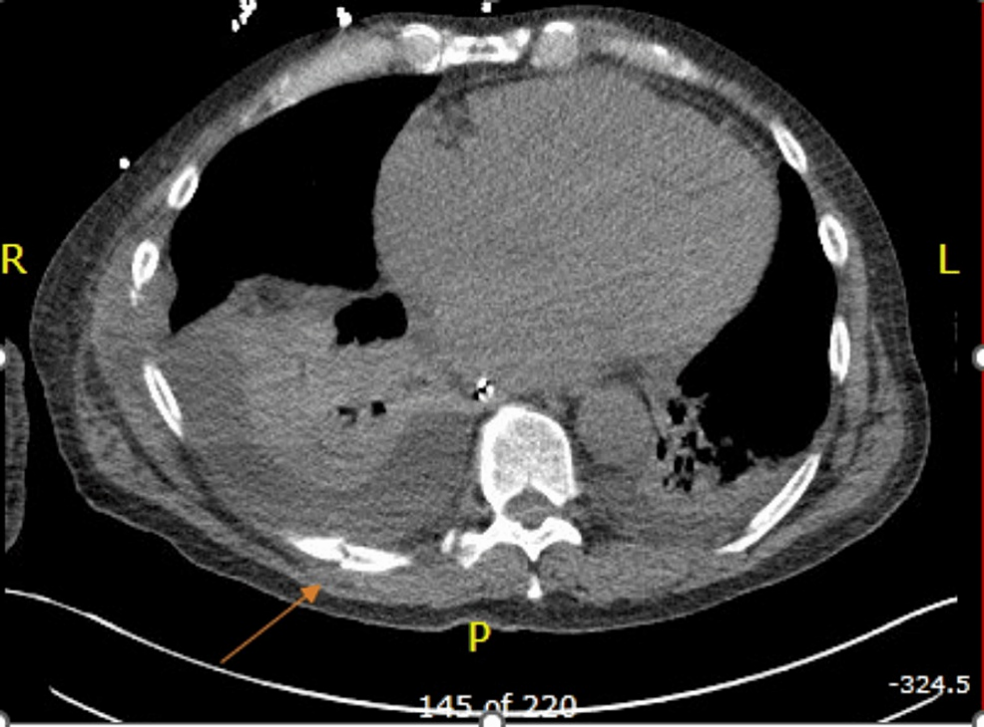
CT chest without contrast CT chest showing right acute rib fracture and small right pleural effusion.
